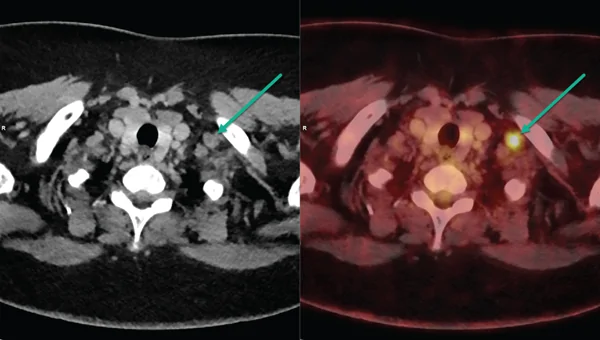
Axial CT and fused 18F‐FDG PET/CT images showing intense FDG uptake in the enlarged left supraclavicular lymph node.
